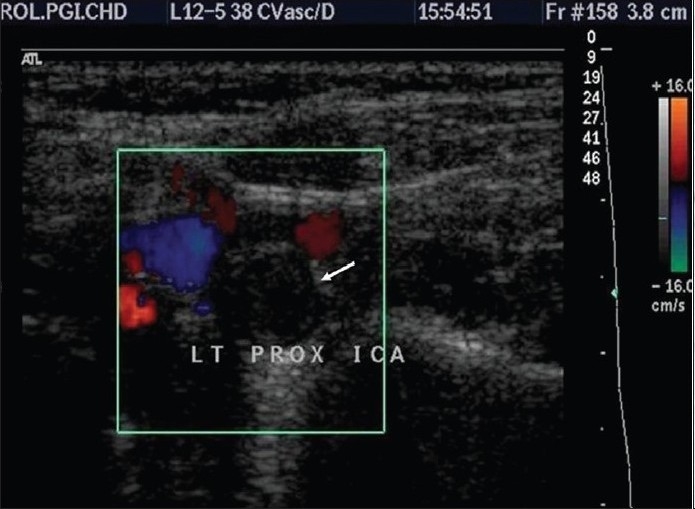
Color doppler (Transverse) showing occluded left ICA
